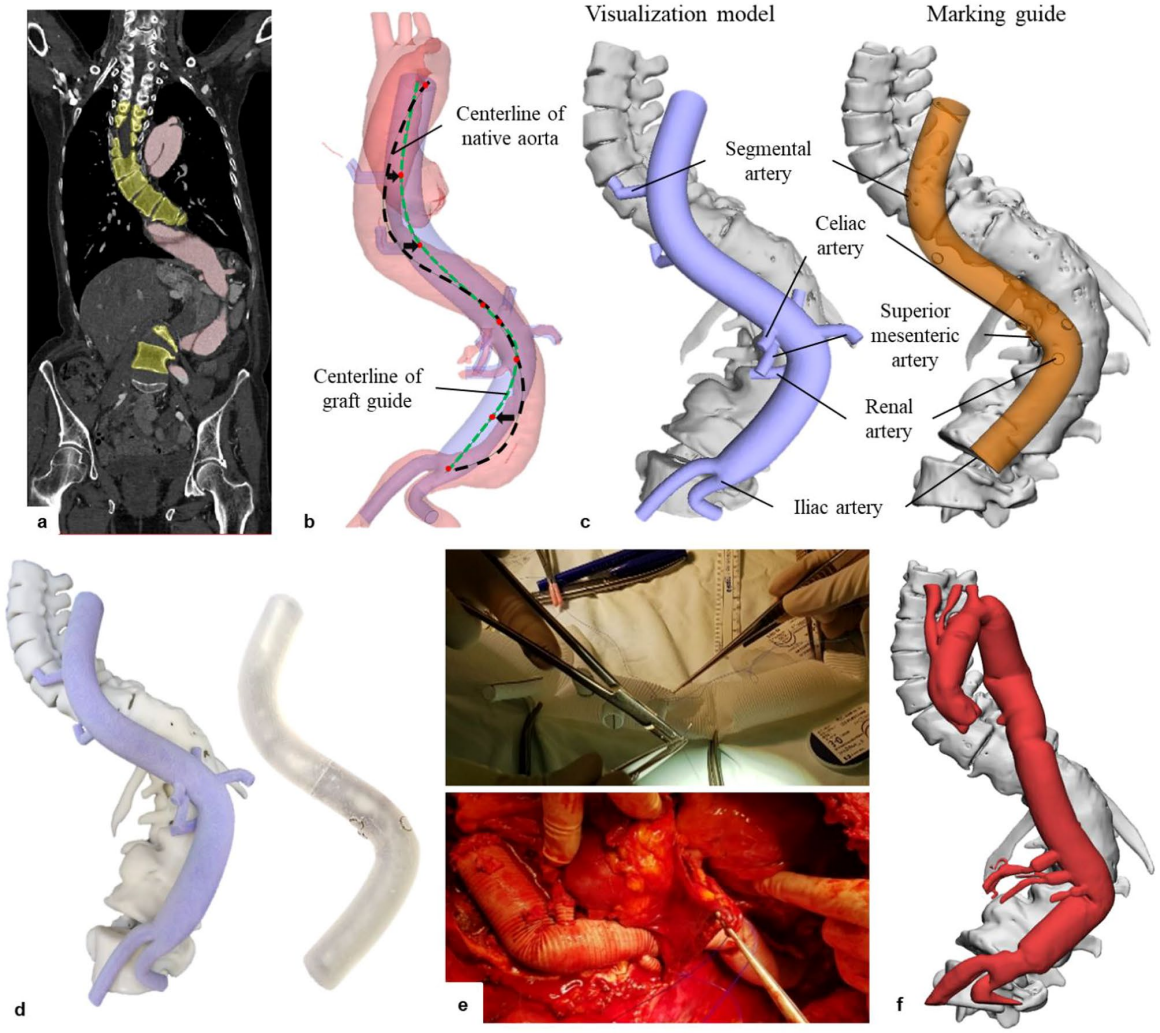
Two types of patient-specific graft reconstruction guide application for open repair of thoracoabdominal aortic aneurysm. (a) CT angiography images and segmentation of diseased aorta and spine; (b) modelling the centreline of graft based on that of a native aorta; (c) 3D modelling of the visualization model and marking guide; (d) 3D-printed visualization model and marking guide; (e) graft reconstruction and clinical application in operating room; (f) postoperative graft.
